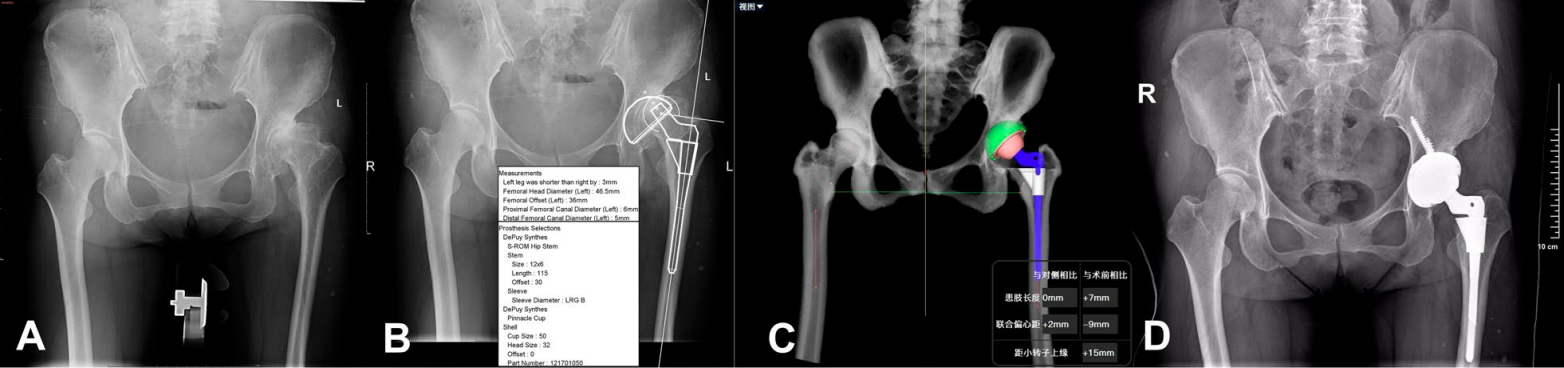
Case 2, woman, 51 years old, Crowe II DDH, left. A Standard preoperative anteroposterior X-ray of the pelvis. B Preoperative planning by the 2D template; planning results: Cup size was 50 mm. C Preoperative planning by AIHIP; planning results: Cup size was 52 mm. D Standard postoperative anteroposterior X-ray of the pelvis: Cup size was 52 mm
